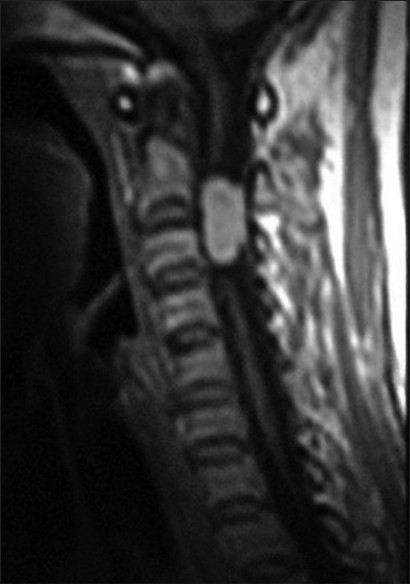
Hyperintense well-defined mass at the C2-C3 level of cervical spinal cord with wide dural attachment on T1W images of the MRI scan
